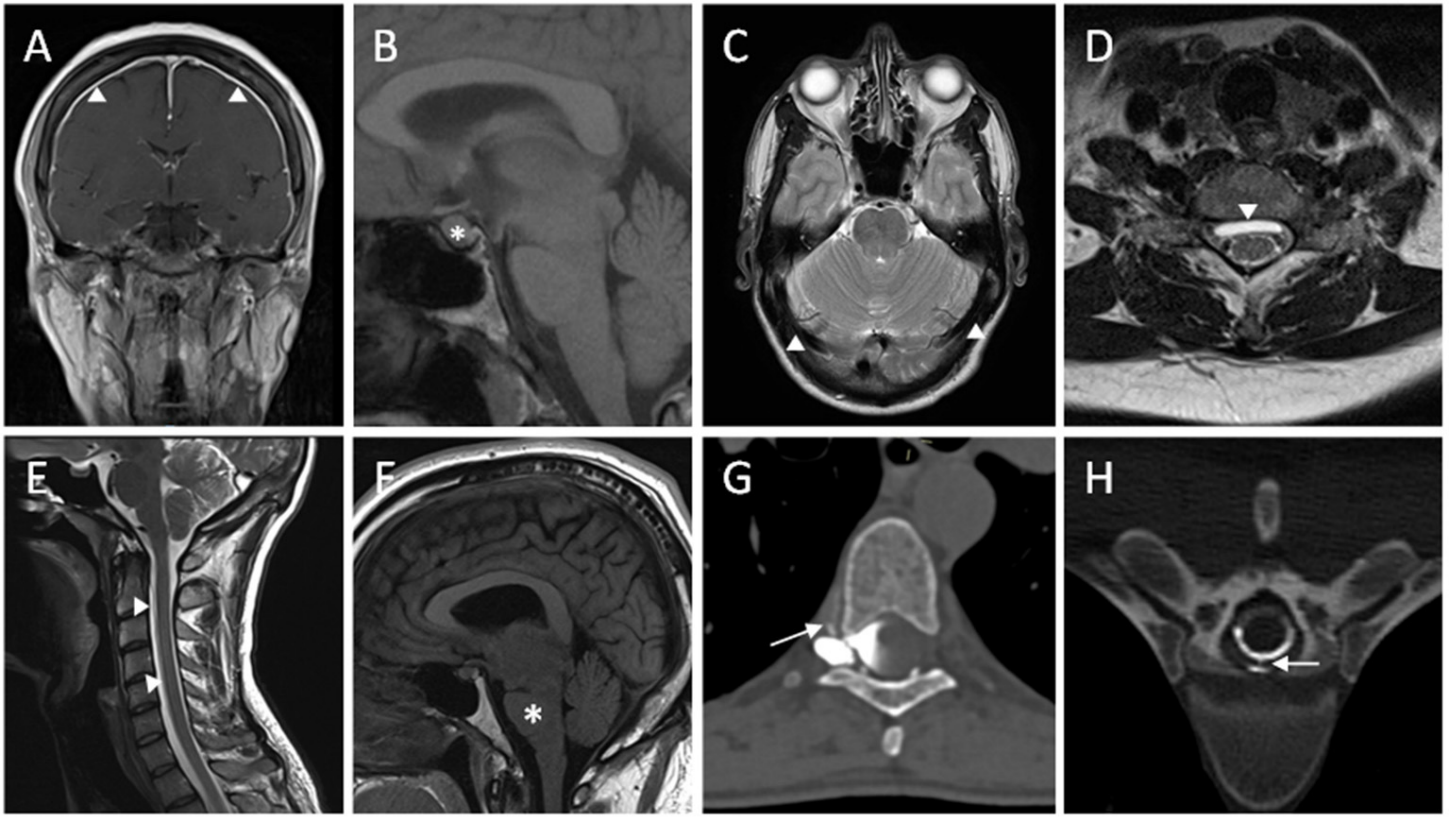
Radiological features of spontaneous intracranial hypotension. (A) T1W coronal post-contrast MRI sequence showing diffuse smooth pachymeningeal thickening and enhancement. (B) Enlargement of pituitary gland seen on T1W sagittal MRI sequence. (C) T2W axial MRI sequence showing distension of the transverse venous sinuses. (D) Ventral extradural CSF collection seen on T2W axial MRI sequence. (E) White arrowheads highlighting a ventral longitudinal extradural CSF collection (SLEC) in the cervical spine on T2W sagittal MRI sequence. (F) T1W sagittal MRI sequence demonstrating brainstem sagging. (G) A right-sided thoracic CSF venous fistula shown with contrast extravasation into a paraspinal vein on right decubitus CT myelography. (H) Extravasation of CSF through a ventral dural tear on CT myelography.
